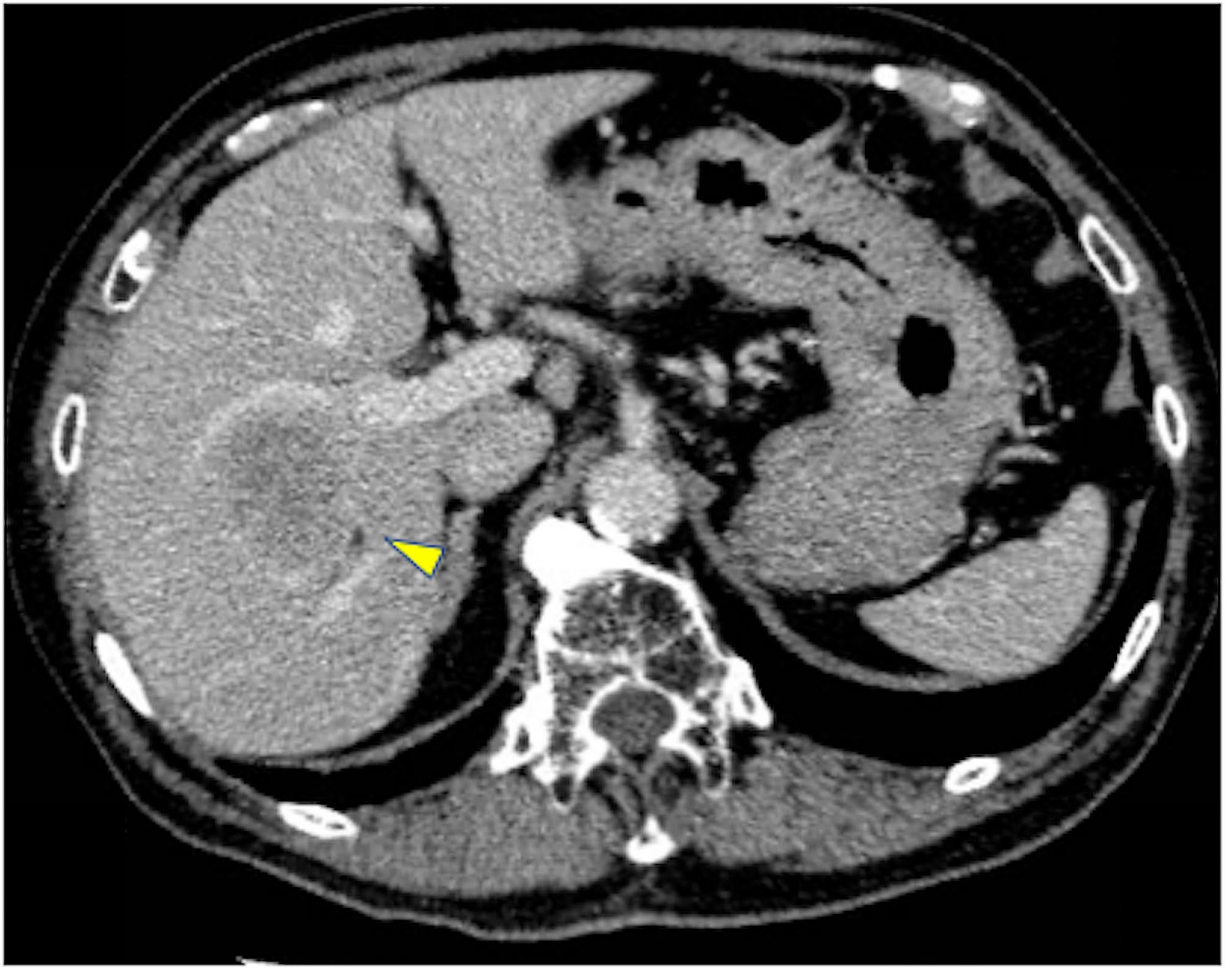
Enhanced abdominal computed tomography (CT) results. Enhanced CT shows a large solitary tumor measuring 6.0 cm located near the bifurcation of the anterior and posterior hepatic branches (arrowhead). CT: computed tomography
